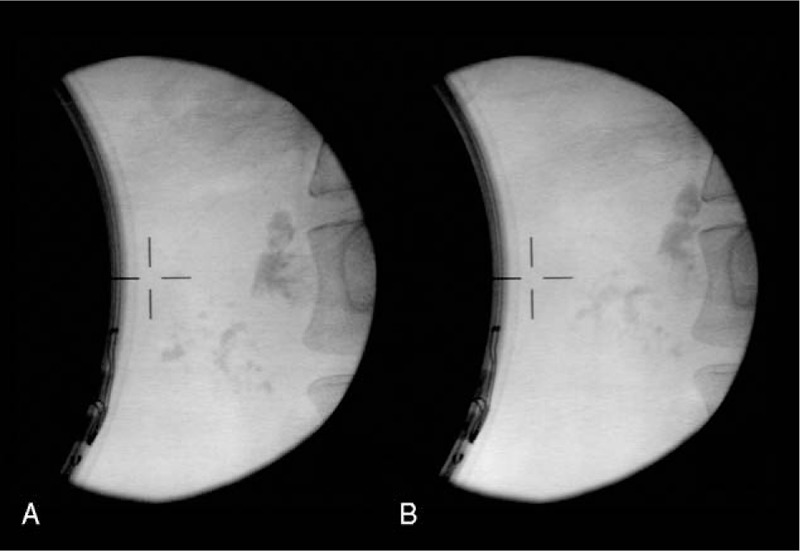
(A) The x-ray image for pancreatic stone of the patient before the first session of extracorporeal shock wave lithotripsy for pancreatic stones (P-ESWL). (B) The x-ray image for pancreatic stone of the patient after 3 sessions of P-ESWL. P-ESWL =  pancreatic extracorporeal shockwave lithotripsy
